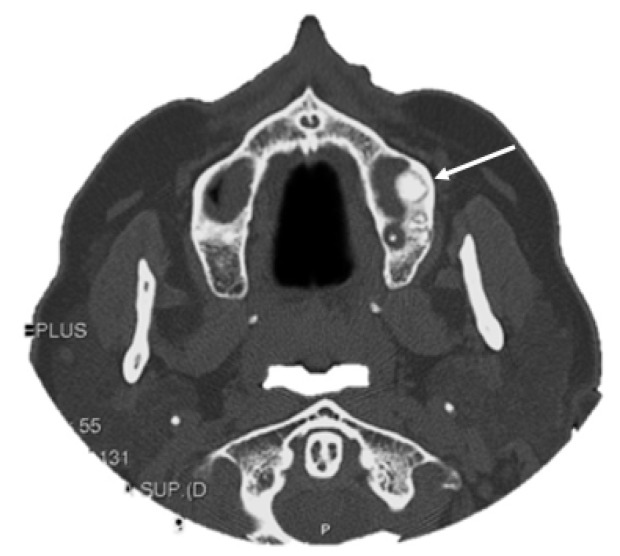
CT scan showing the incidental finding of a small osteoma located in the floor of the left maxillary sinus (white arrow).
